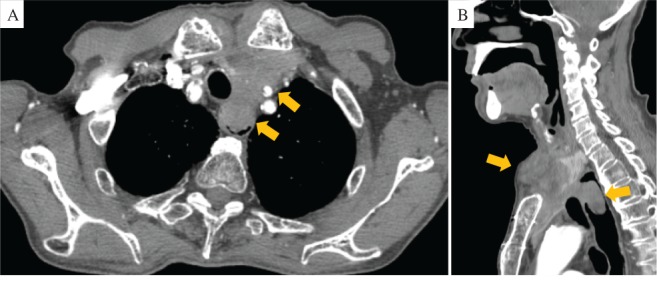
Recent chest computed tomography showed that the mass progressed rapidly and extended into the oesophageal lumen.
